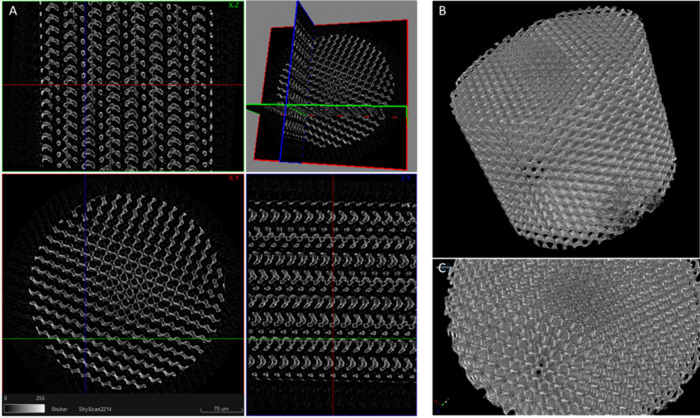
Nano-CT analysis of internal structural
